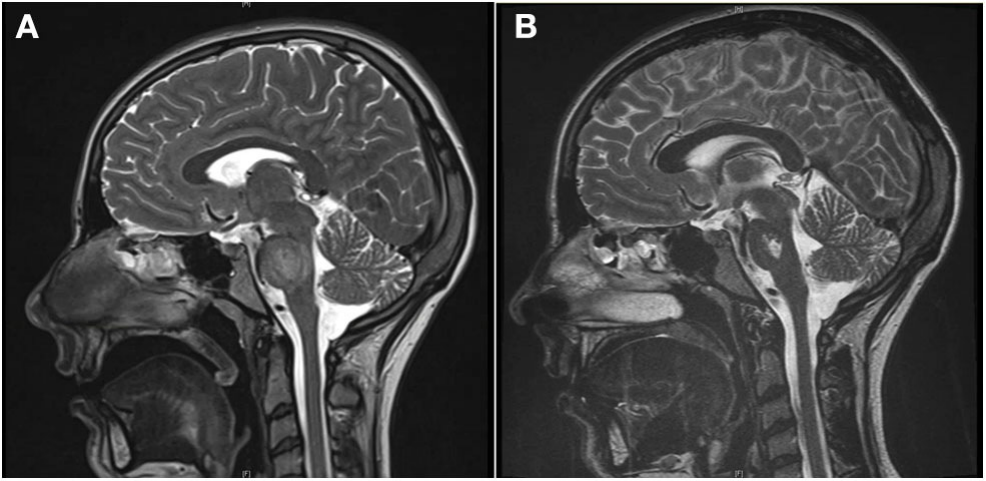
(A) The T2-weighted magnetic resonance tomography shows a diffuse increase of signal intensity and edema in the pons during the acute phase of central pontine myelinolysis. (B) Four weeks later, the edema has resolved, but a trident-like substance defect in the pons remains (T2 weighting).
